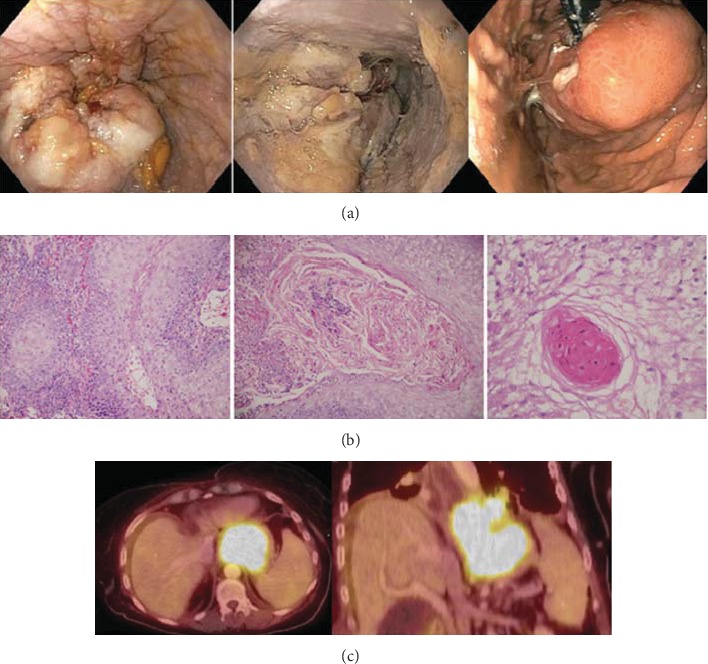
Findings pretreatment. (a) Shows endoscopic views of large, friable, exophytic, and ulcerating mass covered with white exudates in the lower third of the esophagus, gastroesophageal junction, and cardia. (b) Shows pathologic features including (1) a hollow “burrow” with a deep pushing pattern (200x) (2) cyst-like squamous nest filled with keratin and acute inflammatory cells (200x) and (3) keratin pearl in a nest of invasive squamous epithelium. (c) PET/CT shows a large mass in the distal esophagus and proximal stomach without evidence of surrounding adenopathy with mild dilation and likely partial disruption of the distal esophagus.
